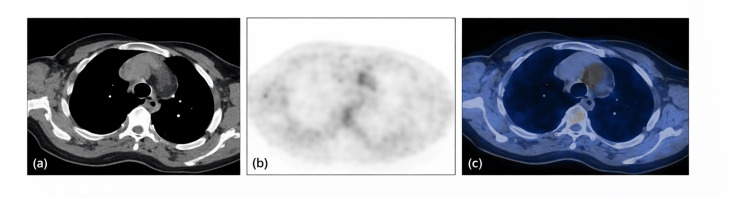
Fluorodeoxyglucose (FDG) PET/CT images at the level of the thoracic aorta. (a) Transaxial CT image depicting the anatomical details of the mediastinum. (b) Corresponding transaxial FDG-PET image showing metabolic tracer distribution. (c) Fused transaxial FDG-PET/CT image demonstrating increased FDG uptake along the aortic wall, consistent with inflammatory involvement of the vessel wall.
